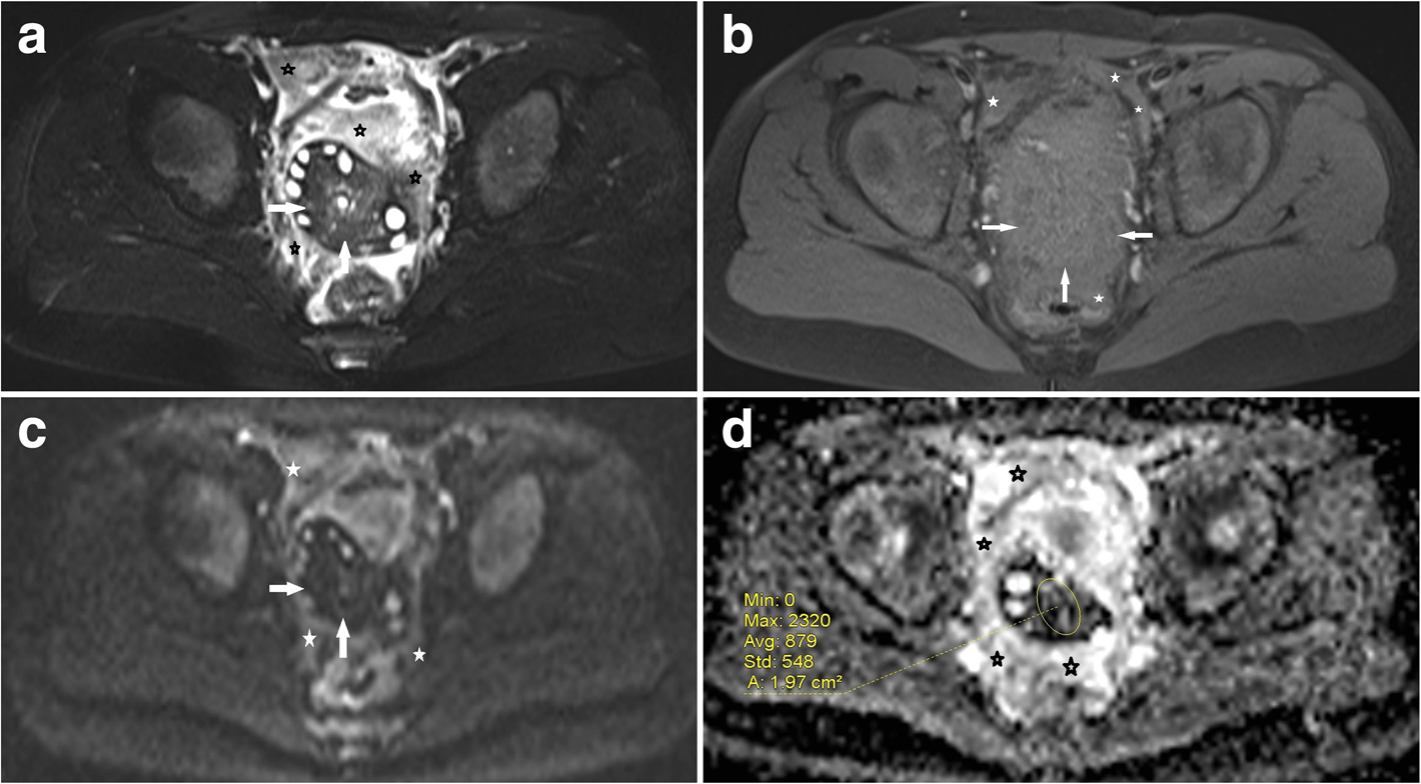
A female patient with right lower quadrant pain for three days. She had surgically confirmed haemorrhagic infarction without any space occupying lesion, and oophorectomy was performed. a T2-weighted fat-suppressed axial image shows an enlarged right ovary with strong stromal hypointensity (arrows) and peripherally deplased follicles. b T1-weighted fat-suppressed axial image reveals slight hyperintensity of swollen stroma compared to iliopsoas muscle (arrows). Note also the slightly hyperintense haemorrhagic free peritoneal fluid in the pelvic resseses (stars). c b 1000 image shows strong hypointensity in the ovarian stroma (arrows) and hyperintense haemorrhagic fluid in the pelvic resseses (stars). d Visual assessment of ADC map shows strong stromal hypointensity (ADC value, 0.87 × 10^−3^ mm^2^/s) and hypointense haemorrhagic fluid in the pelvic resseses compared to urinary bladder
